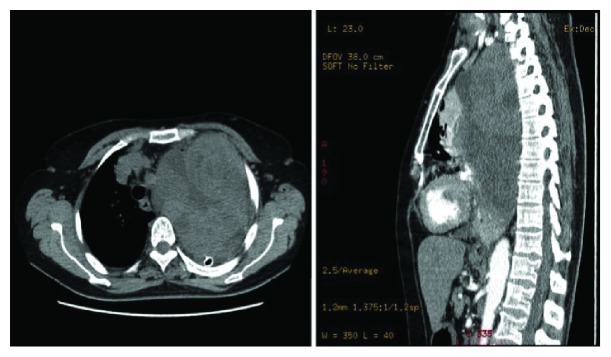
Chest computed tomography scan of an intrapulmonary solitary fibrous tumor, largely occupying the left hemithorax (mediastinal window).
